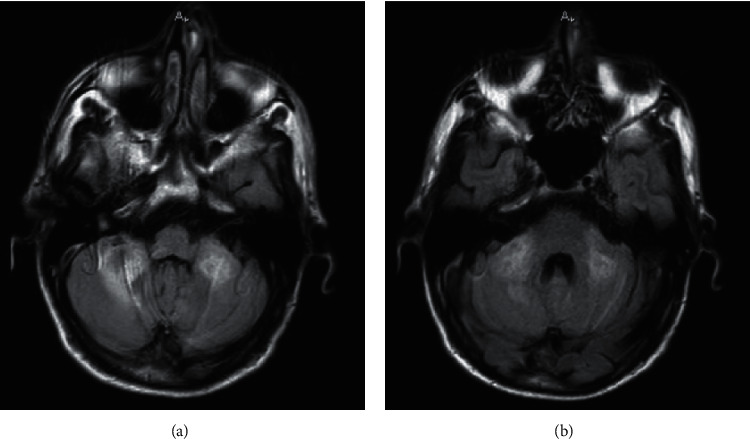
(a, b) Two consecutive axial fluid attenuation inversion recovery sequence images of brain MRI without contrast from the posterior fossa. Increased signal intensity is evident in bilateral cerebellar peduncles.
